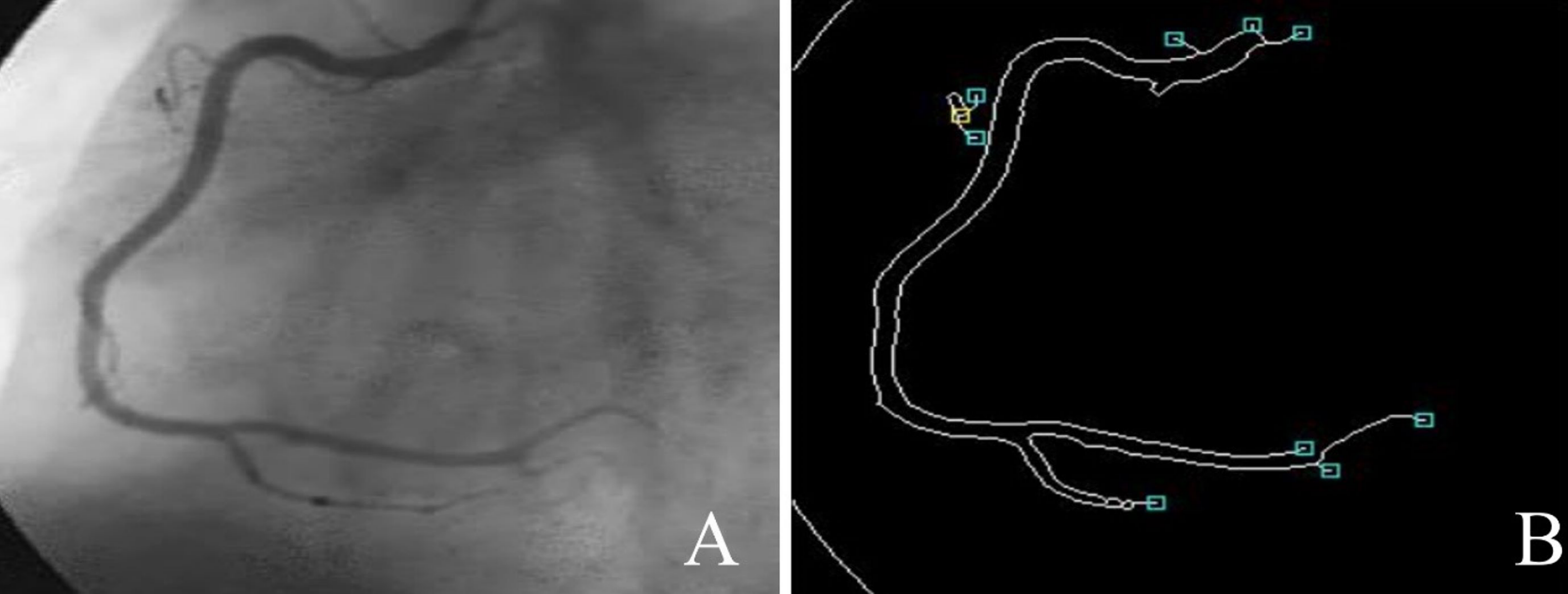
(A) X-ray angiography, (B) Segmented image with stenosis.
